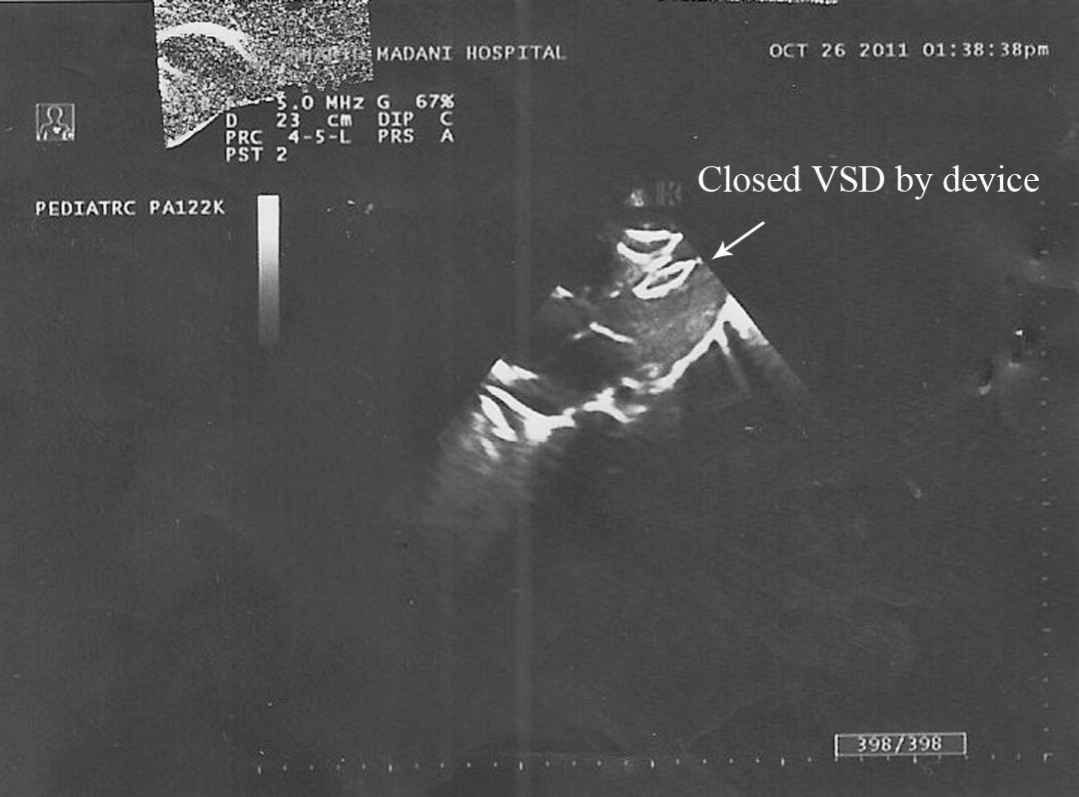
Apical 4-chamber view by epicardial echocardiography shows the device at the position of apicomuscular defect after defect closure via the periventricular procedure.
